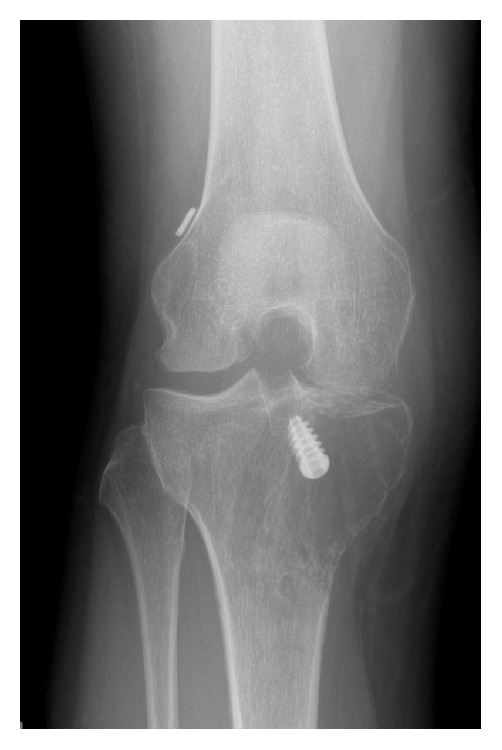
AP radiograph of the right knee shows significant progression of the tibial plateau osteolytic lesion in the medial tibial plateau, which now extends to the epiphysis.
